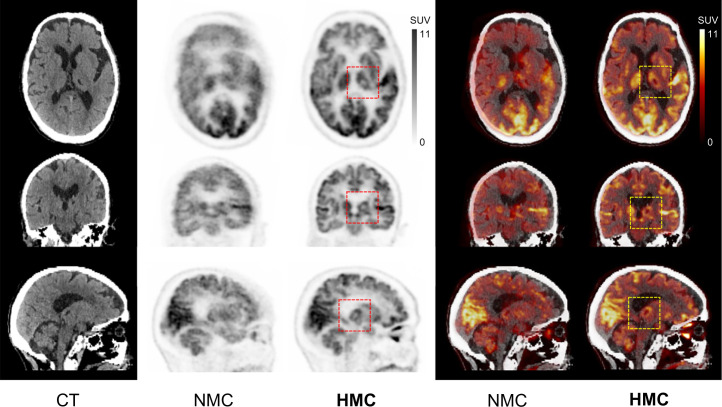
CT, PET, and PET/CT with HM before and after HMC in case of focal hypometabolism observed in left thalamic and basal ganglia region after HMC. Injected dose was 229.4 MBq, postinjection time was 69 min, frame duration was 3 min, and body weight was 48 kg. Averaged and maximal HM distance of frontal lobe was 13.7 and 25.4 mm, respectively.
